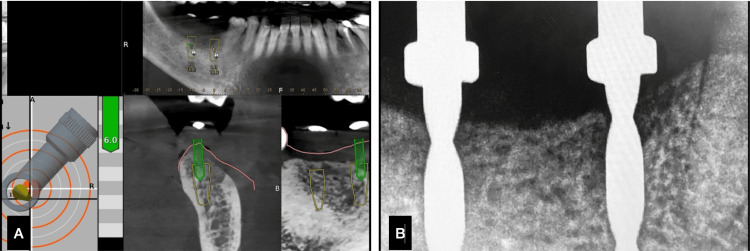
Case 2. A) Drilling under Dynamic navigation, B. position indicating device (PID) radiograph.
